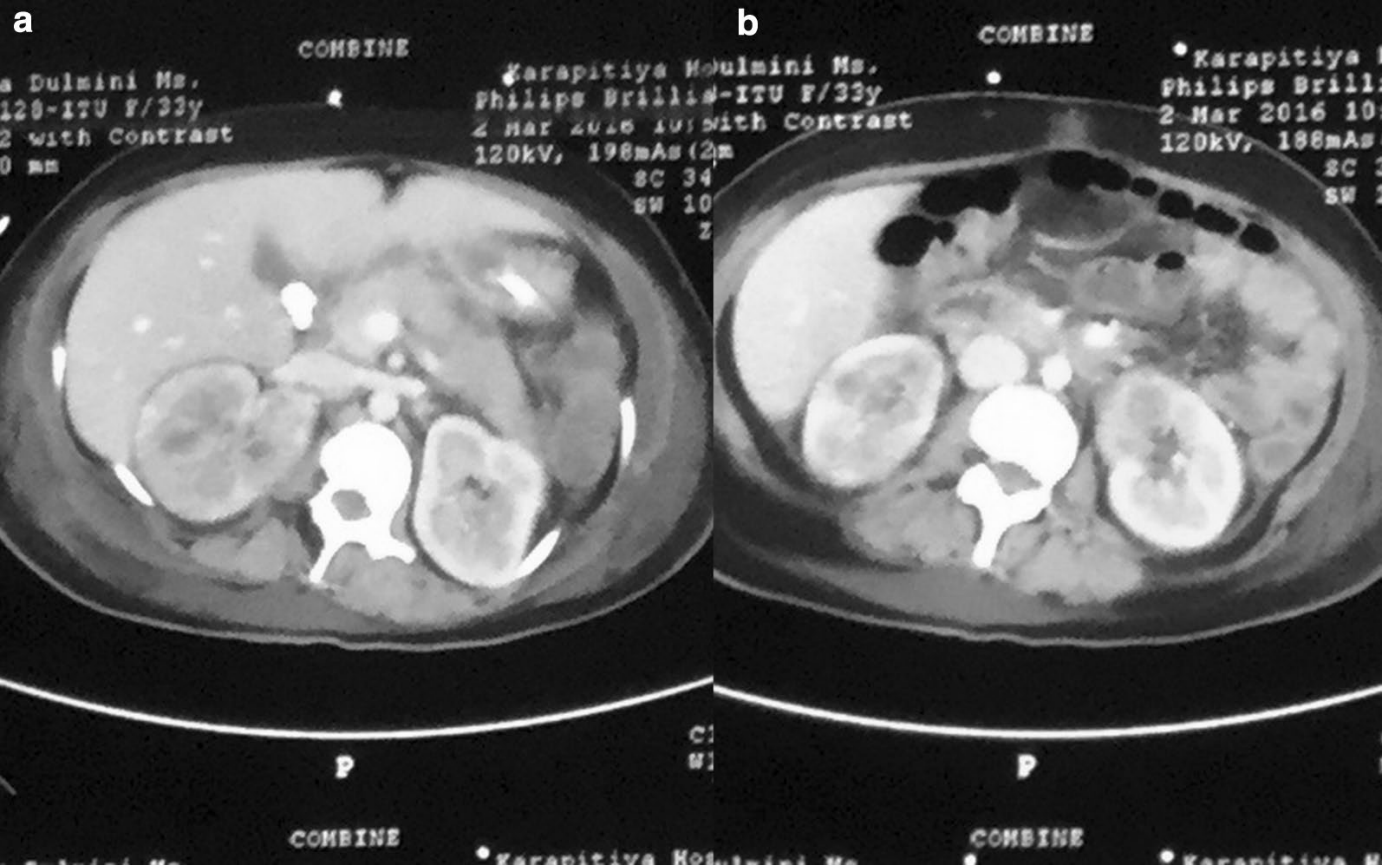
Contrast enhanced computed tomography of abdomen. Showing bilateral pyelonephritis with abscess on right upper pole (a) and early abscess formation on left lower pole (b)
